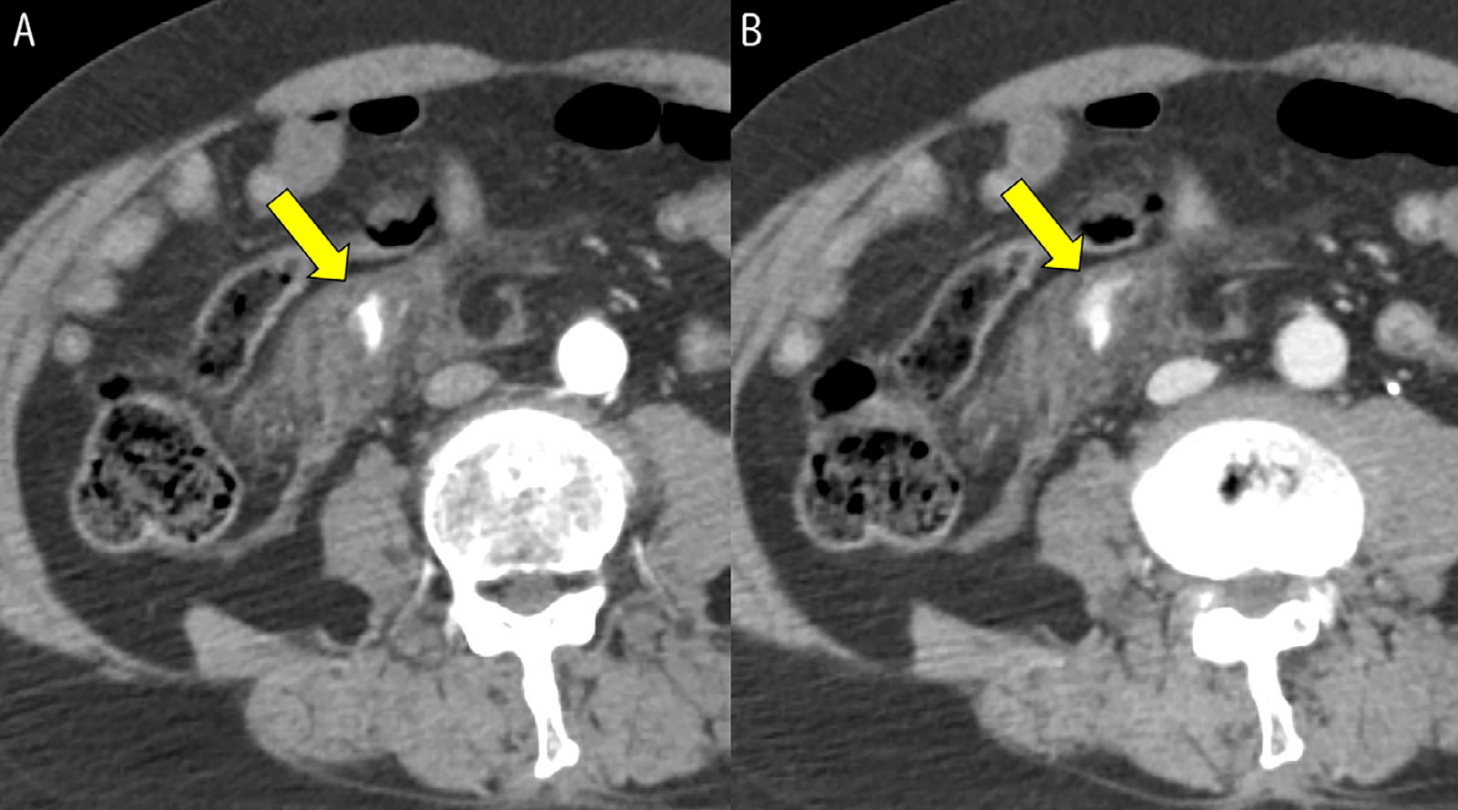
Abdominal contrast-enhanced CT. (A) arterial phase; (B) equilibrium phase (B is the same level as A) Contrast-enhanced computed tomography (CT) revealed an isolated mesenteric hematoma with contrast extravasation in the territory of the ileocolic artery in the right lower abdomen during the arterial phase (A, arrow). The contrast material spread further in the equilibrium phase (B, arrow).
